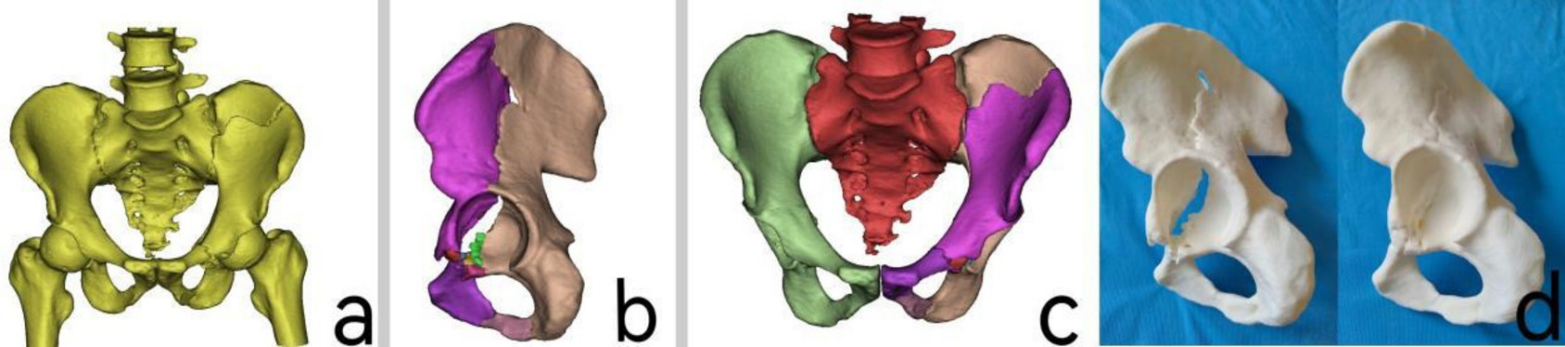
a: Three-dimensional modeling of the patient’s pelvis using Mimics software based on CT imaging data. b: Removal of the femur and lumbar vertebrae, followed by separation of the fracture fragments using the threshold selection function, resulting in the three-dimensional modeling of the patient’s pelvic fracture. c: Anatomical virtual reduction of the fracture fragments to obtain the three-dimensional model of the pelvis after reduction. d: Printing of life-sized 1:1 scale fracture physical models and fracture physical models after virtual reduction.
